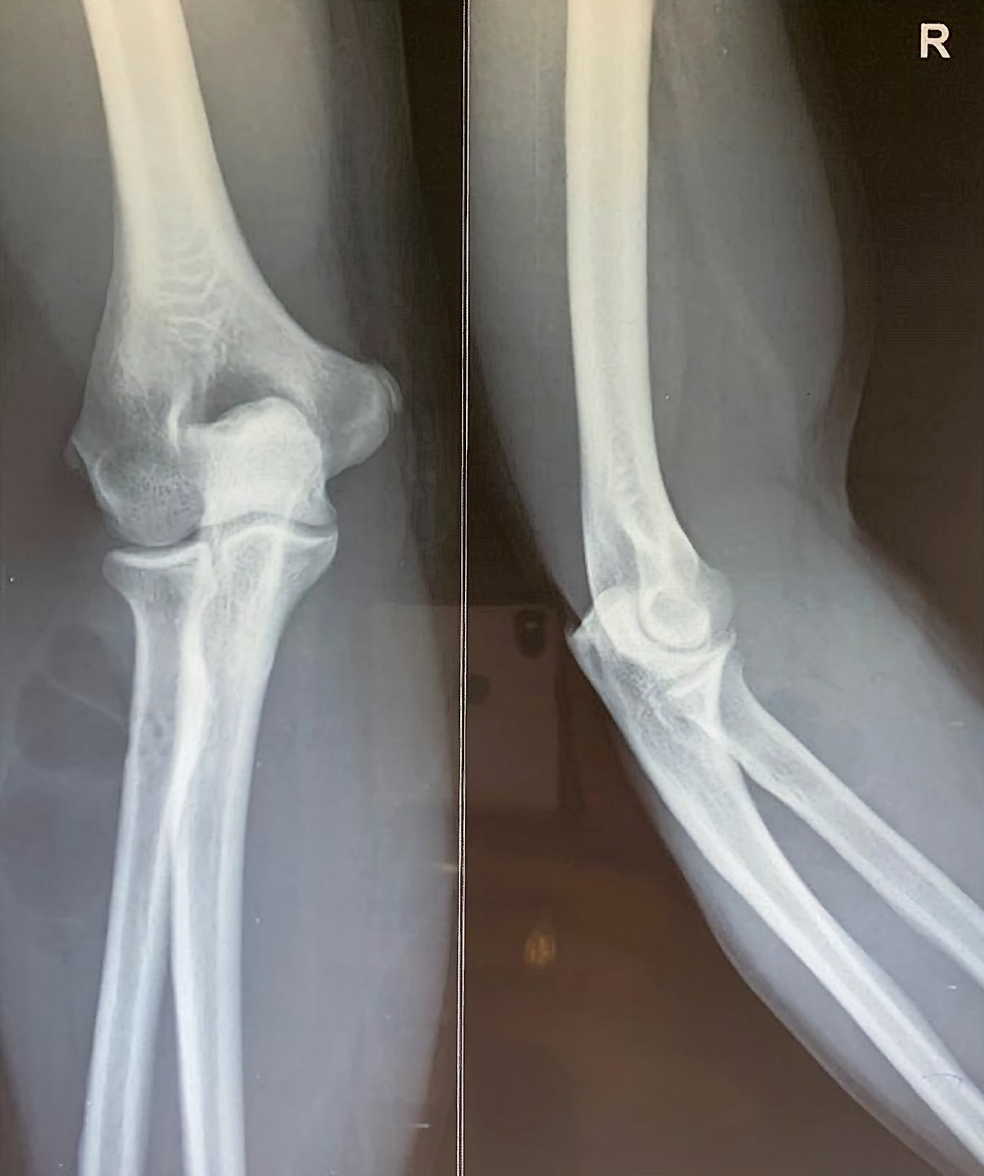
Plain X-ray of the right elbow with the forearm
